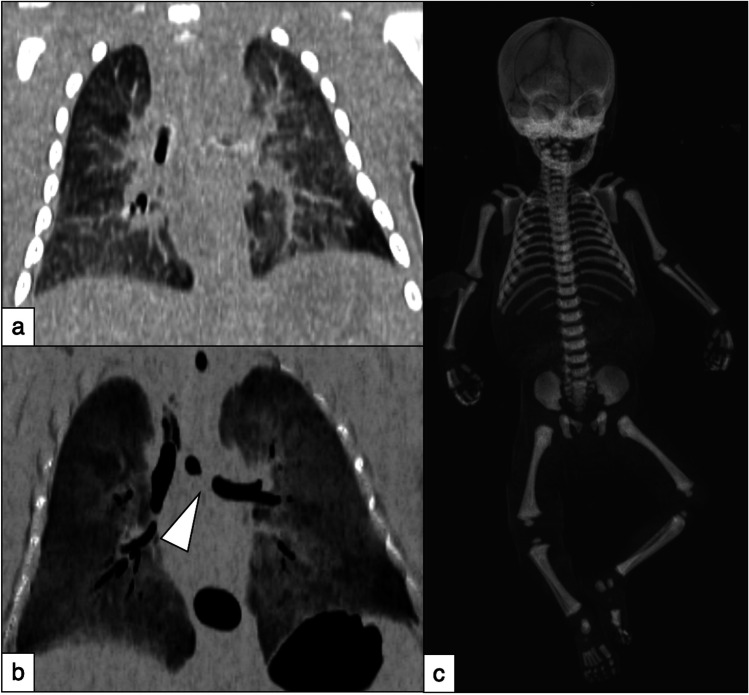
Post-mortem non-contrast CT. (a) Reconstructions at maximum intensity projection (4 mm thickness – coronal view) show diffuse inhomogeneity on both lungs with a diffuse ground glass opacifications and tiny air space consolidations as for alveolar oedema. (b) Chest details: reconstructions at minimum intensity projection (4 mm thickness – coronal view) confirm a diffuse ground glass opacifications on both lungs. Left main bronchus is partially occluded by mucoid secretions (white arrowhead). (c) Volume rendering reconstructions: no signs of traumatic and/or pathological fractures
